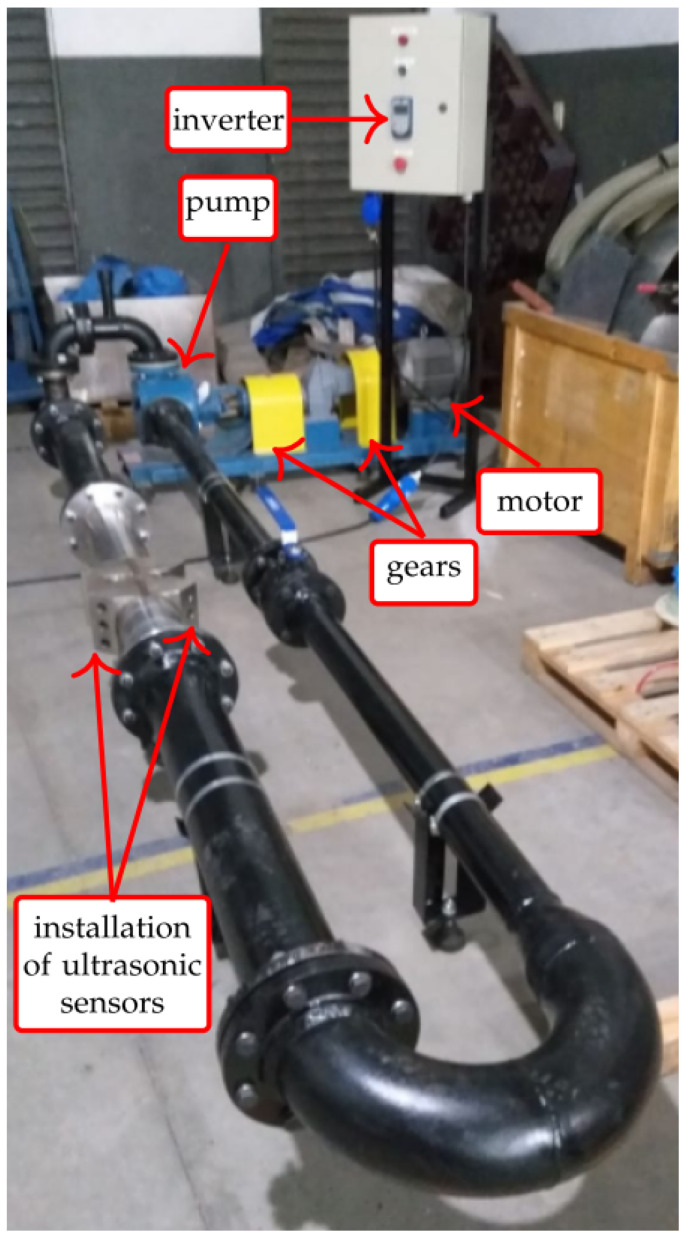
Evaluation setup for the ultrasonic sensors.
